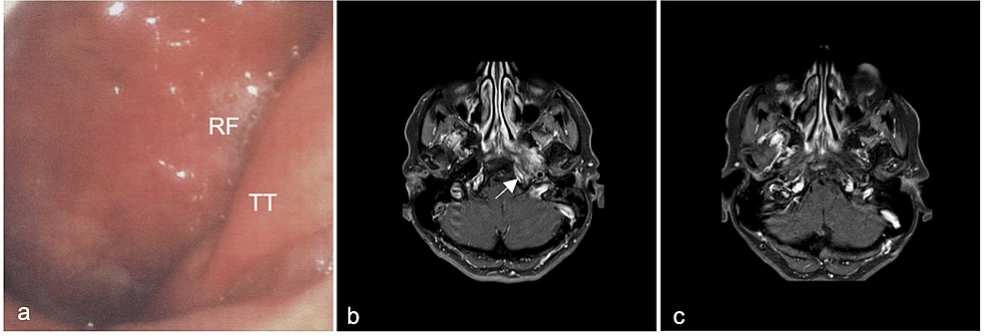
Endoscopic examination of nasopharynx, T1-weighted MRI imaging, and radiological findings after treatment. (a) Diffuse, bulging erythema of the left nasopharynx as initial finding upon endoscopic examination of nasopharynx. (b) Contrast-enhanced, T1-weighted MRI imaging, axial projection of the mass (white arrow). The mass was extending to the foramen lacerum and ovale and was surrounding the carotid canal and the Eustachian tube. The clivus was also partially infiltrated on the left side. Fluid retention in the left mastoid cavity and cervical/retropharyngeal lymphadenopathy was noted. (c) Almost complete resolution of radiological findings after treatment. TT: torus tubarius; RF: Rosenmüller’s fossa
